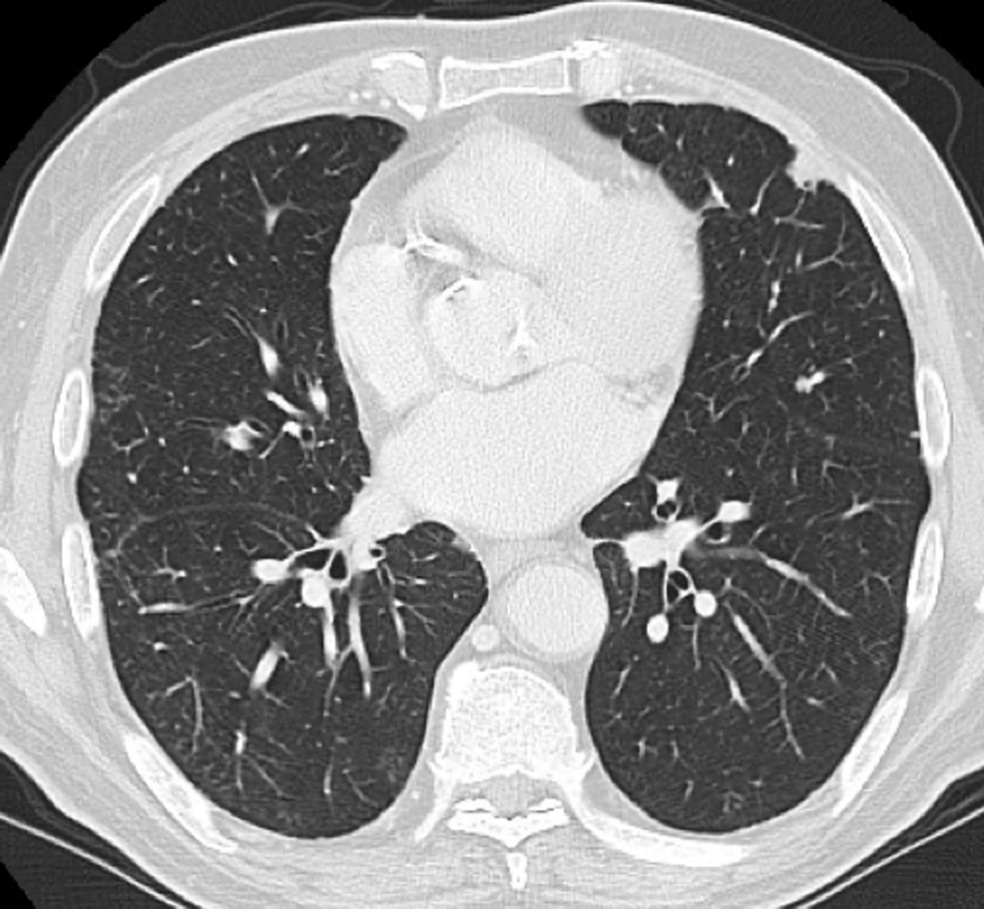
A reassessment CT scan done after 12 cycles of chemotherapy showing stable metastatic disease (Case 4). CT chest showing response to treatment.
